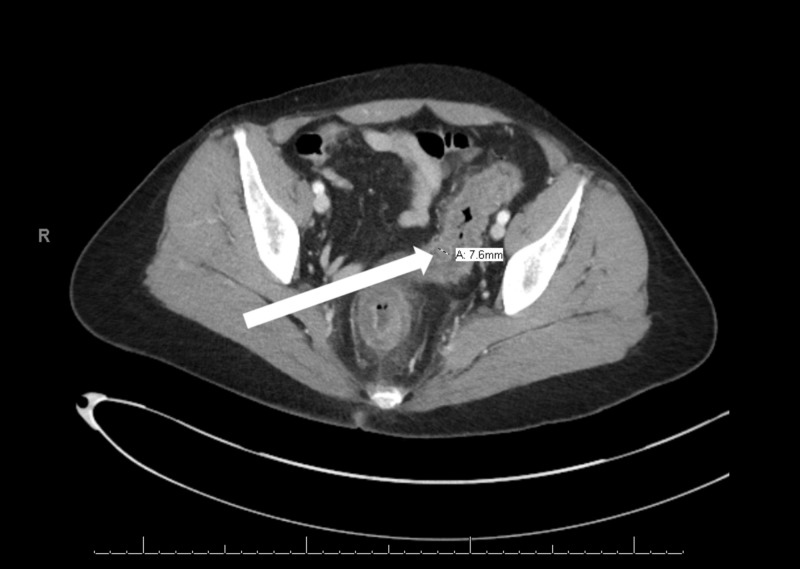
CT abdomen and pelvis with contrast scan showing the anal canal and entire sigmoid colon with circumferential wall thickening measuring up to 7 mm (arrow) with minimal adjacent fat stranding without focal collection of free air.
